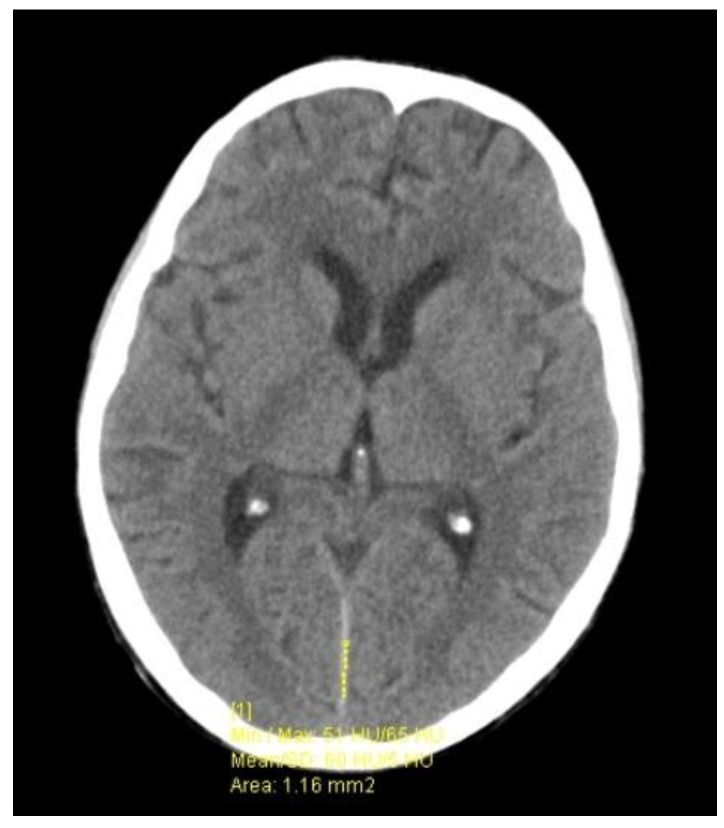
Control head CT. No parenchymal lesions. Tentorium and falx cerebelli appeared slightly thickened with a density of 61 Hounsfield units (suspicion of a small subarachnoid hemorrhage).
